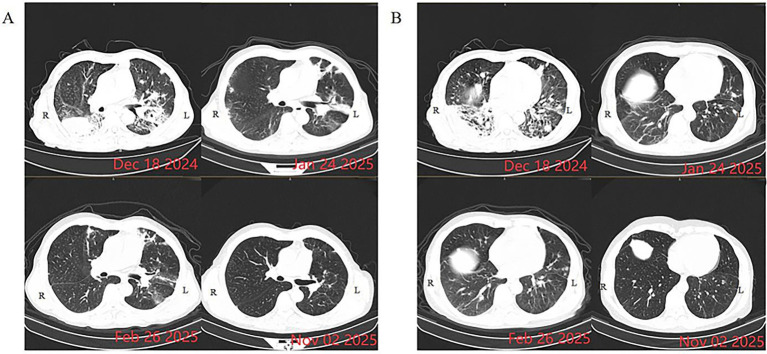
Changes in CT images of the left (A) and right (B) lungs of the patient with invasive pulmonary aspergillosis before and after treatment. On December 18, 2024, the imaging report indicated multiple patchy opacities in both lungs, with small cavity formation in some lesions presenting as air-crescent signs, more prominent in the right lung, suggestive of Aspergillus infection. On January 24, 2025, chest CT showed significant improvement of the infection in the right lung, but progression of the lesion in the left upper lobe, presenting as a large area of high-density opacity. A follow-up CT on February 26 showed improvement of the left upper lobe lesion. On November 2, follow-up imaging showed significant improvement in both lungs.
